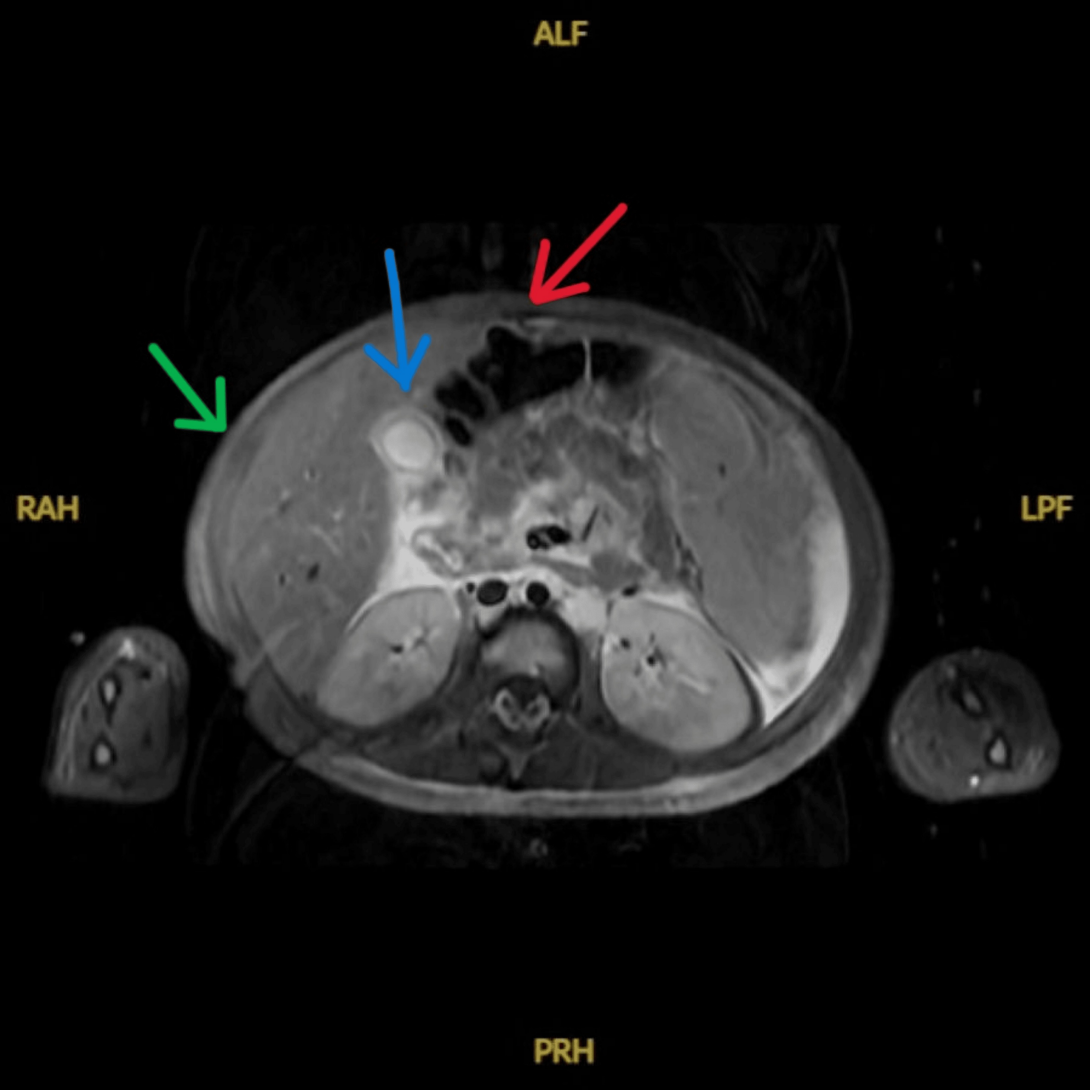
Whole-Body MRI (Axial View) Demonstrating Diffuse Anasarca (Green Arrow), Ascites (Red Arrow), and Gallbladder Edema (Blue Arrow) MRI, magnetic resonance imaging.
